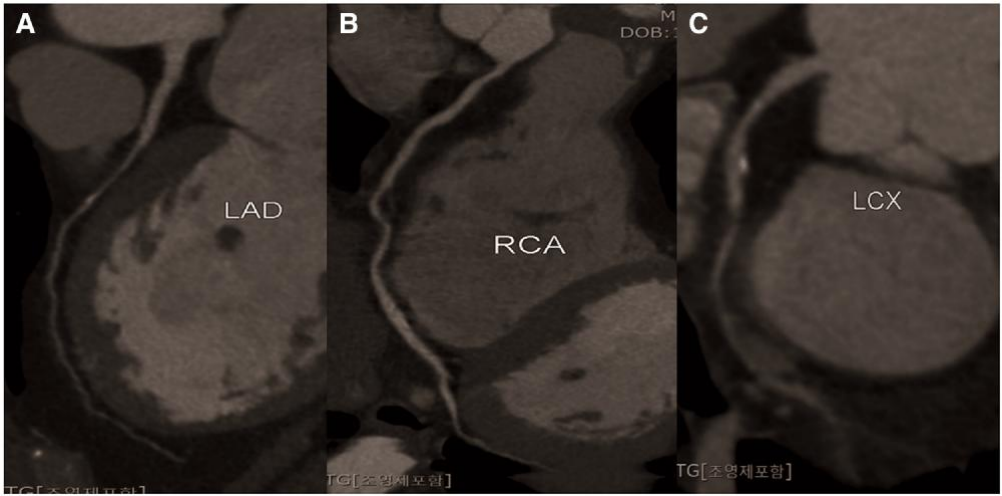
Case 1: curved multiplanar reformation imaging from multi-detector computed tomographic coronary angiography. Baseline computed tomographic imaging reveals diffuse luminal narrowing in multivessel coronary arteries. (*A*) Left anterior descending artery. (*B*) Right coronary artery. (*C*) Left circumflex artery.
